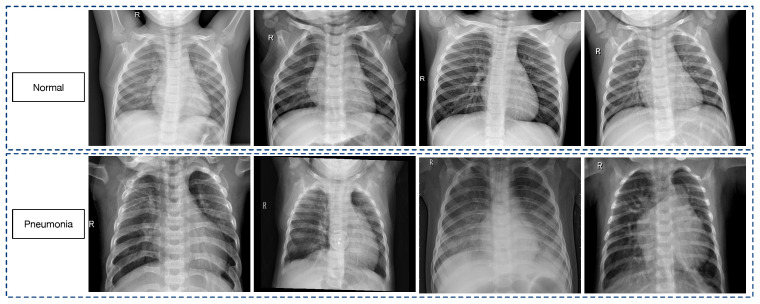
Some samples of the chest X-ray images dataset.
